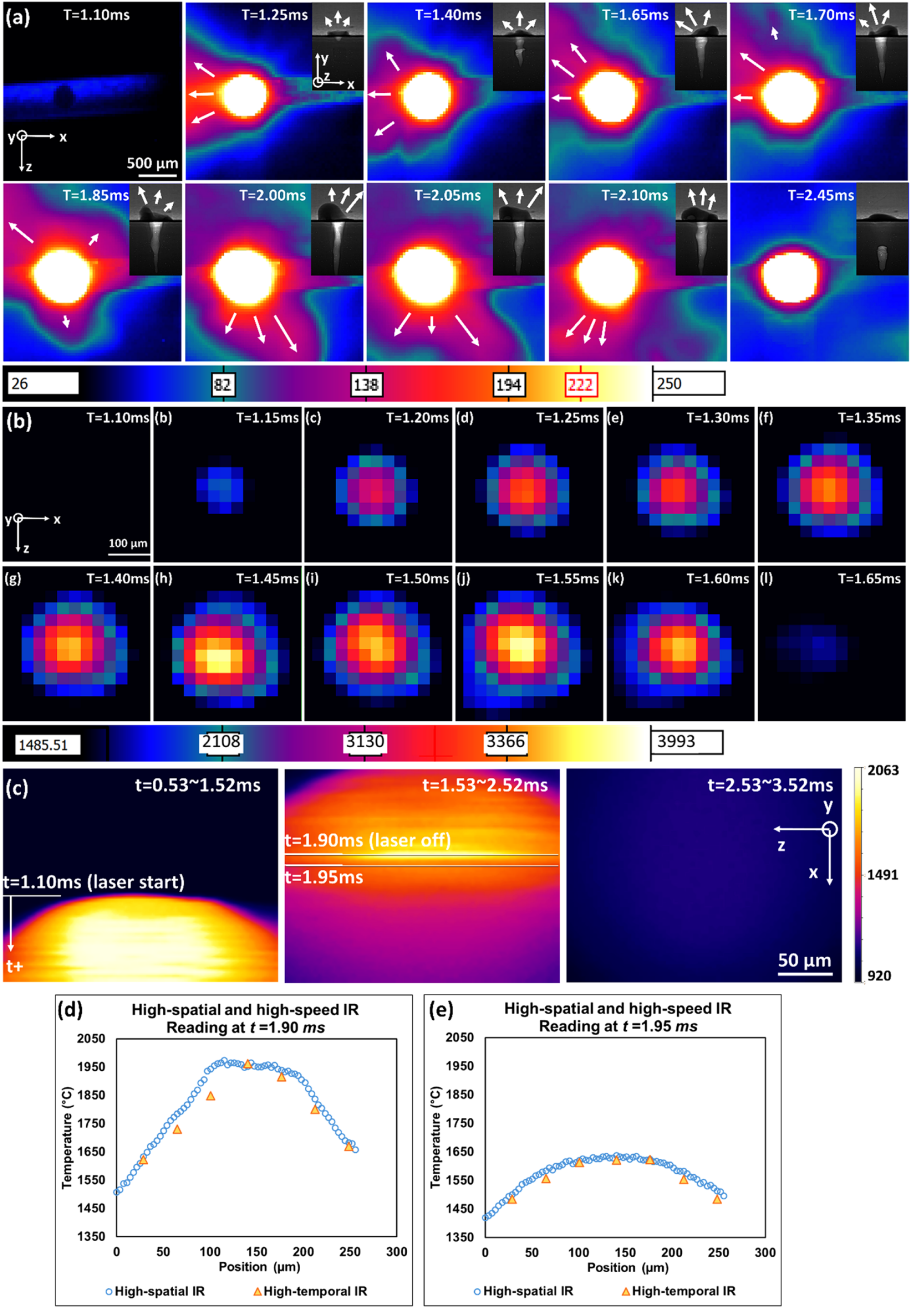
(a) Selected high-speed IR with a low-temperature filter showing vapor plume dynamics of Trial #9 (410 SS, 416 W 1 ms), where the dynamics of the vapor plume can be observed. The corresponding X-ray images in the upper right corner share the same scale as the IR images. (b) Selected high-speed IR with a high-temperature filter, showing melt pool temperature dynamics of Trial #12 (Ti–6Al–4V, 416 W, 0.5 ms). (c) The high-spatial IR observations of Trial #19 (Ti–6Al–4V, 260 W, 0.8 ms). The vertical direction contains both spatial and temporal information due to the camera sensor reading line by line from top to bottom. (d) The high-spatial and high-speed IR readings at the same location of *t* = 1.90 ms. (e) The high-spatial and high-speed IR readings at the same location of *t* = 1.95 ms. (Temperatures all in °C).
